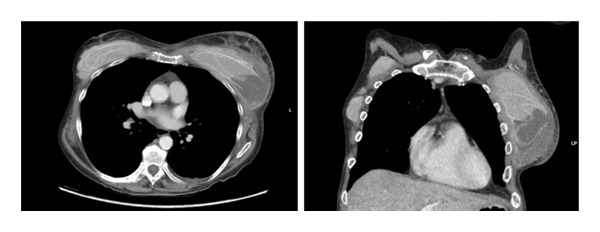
Computed tomography of the chest showing cellulitis, fluid collection, and possible implant rupture.
